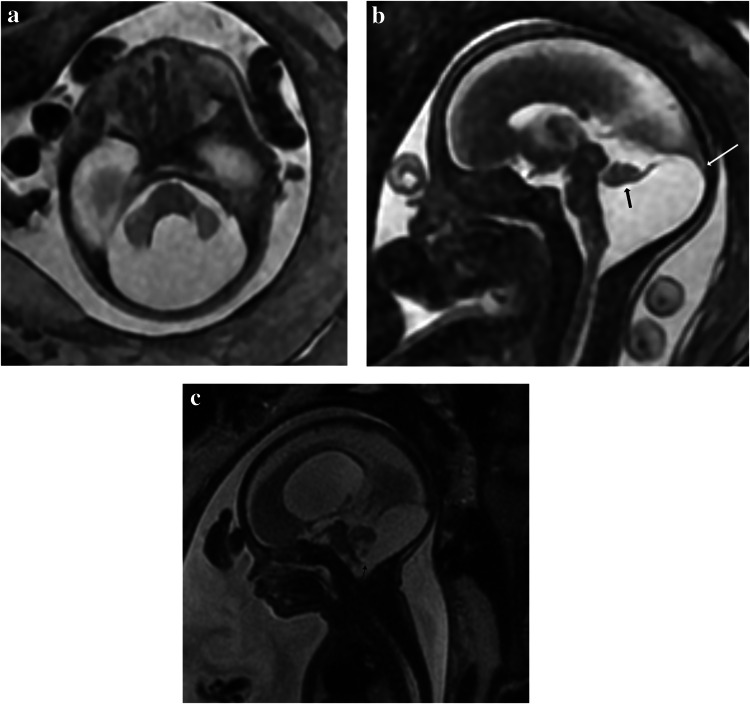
Dandy-Walker malformation (DWM). Fetal brain MRI was performed at 23 0/7 weeks. Axial T2 SSFSE (a) demonstrates pronounced enlargement of the posterior fossa and abnormal CSF space between the cerebellar hemispheres in the axial plane through the mid posterior fossa in place of the expected vermis. On the sagittal T2 fast steady-state image through the midline posterior fossa (b), an abnormally elevated torcula (*white arrow*), small vermis, enlargement of the fourth ventricle with a blunted fastigium (*black arrow*), and communication with the retrocerebellar CSF space are apparent, diagnostic of DWM. c A different case of DWM in a fetus at 21 1/7 weeks, with a sagittal T2 SSFSE off-midline sagittal projection demonstrating the tela choroidea (*black arrow*) positioned ectopically, low and lateral, typical of the Dandy-Walker phenotype, in contrast to high and medial positioning with BPC
